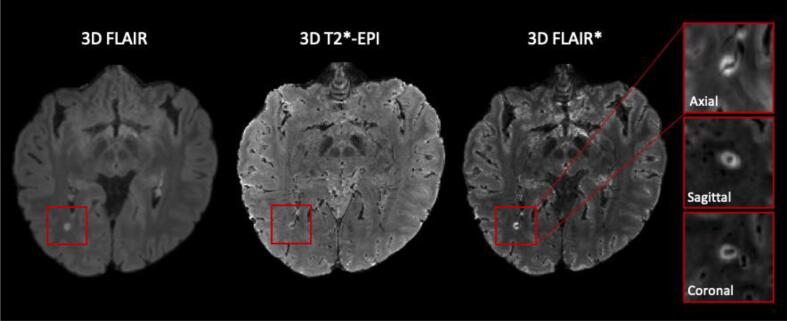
A central vein running through a lesion visible in the three planes (zoomed-in boxes) in a 3D FLAIR* obtained combining FLAIR and T2*-*EPI* acquisitions at 3 T. Resampling was applied to the magnified images for visualization purposes. FLAIR, T2*-*EPI* and FLAIR* are the MRI contrasts that have been used by ML approaches for CVS detection. Refer to the supplementary material for additional examples of the CVS on different susceptibility-weighted imaging sequences.
